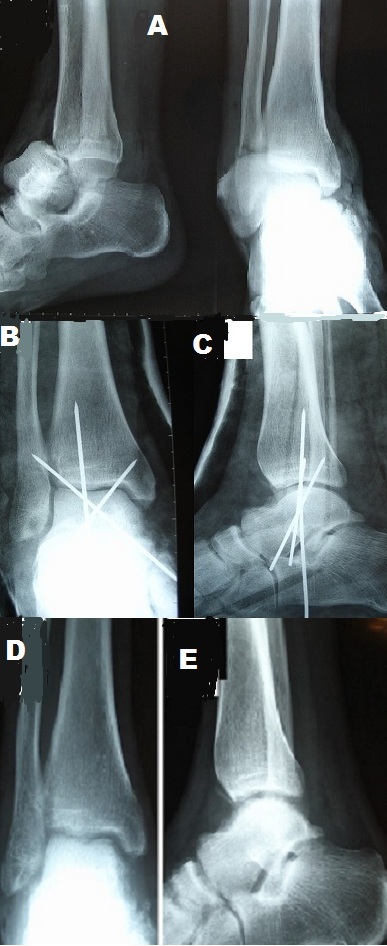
A): the radiography of the ankle objectified anterolateral complete enucleation of the talus with a fracture of the non-displaced lateral malleolus; B, C): the radiography of the ankle objectified trans-calcaneofibular -talo-tibial pin and two Astragalus-tibial pin cross; D,E): the standard clichés of the ankle showed no abnormal bone or joint, or sign of necrosis
